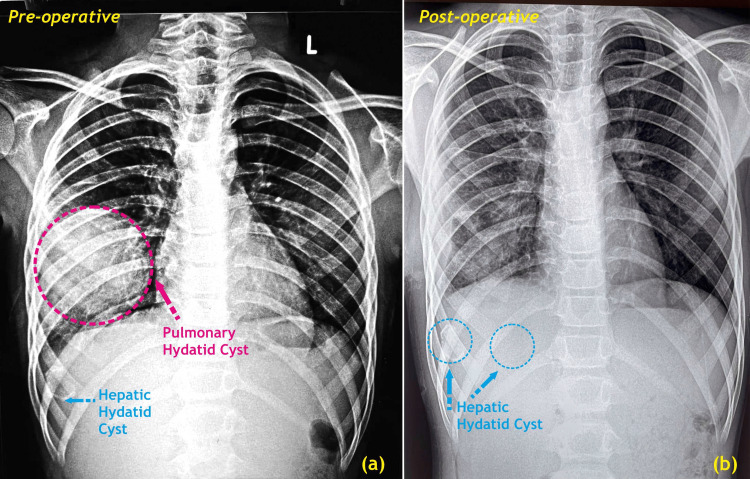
(a) Pre-operative chest X-ray revealing dense homogeneous radio-opaque opacity in the right lower lobe of the lung (red marking) with calcified hepatic hydatid cysts (blue marking); (b) Post-operative chest X-ray without opacity but with persisting hepatic hydatid cysts (blue marking) Image credits: Dr. Vishal V. Bhende
